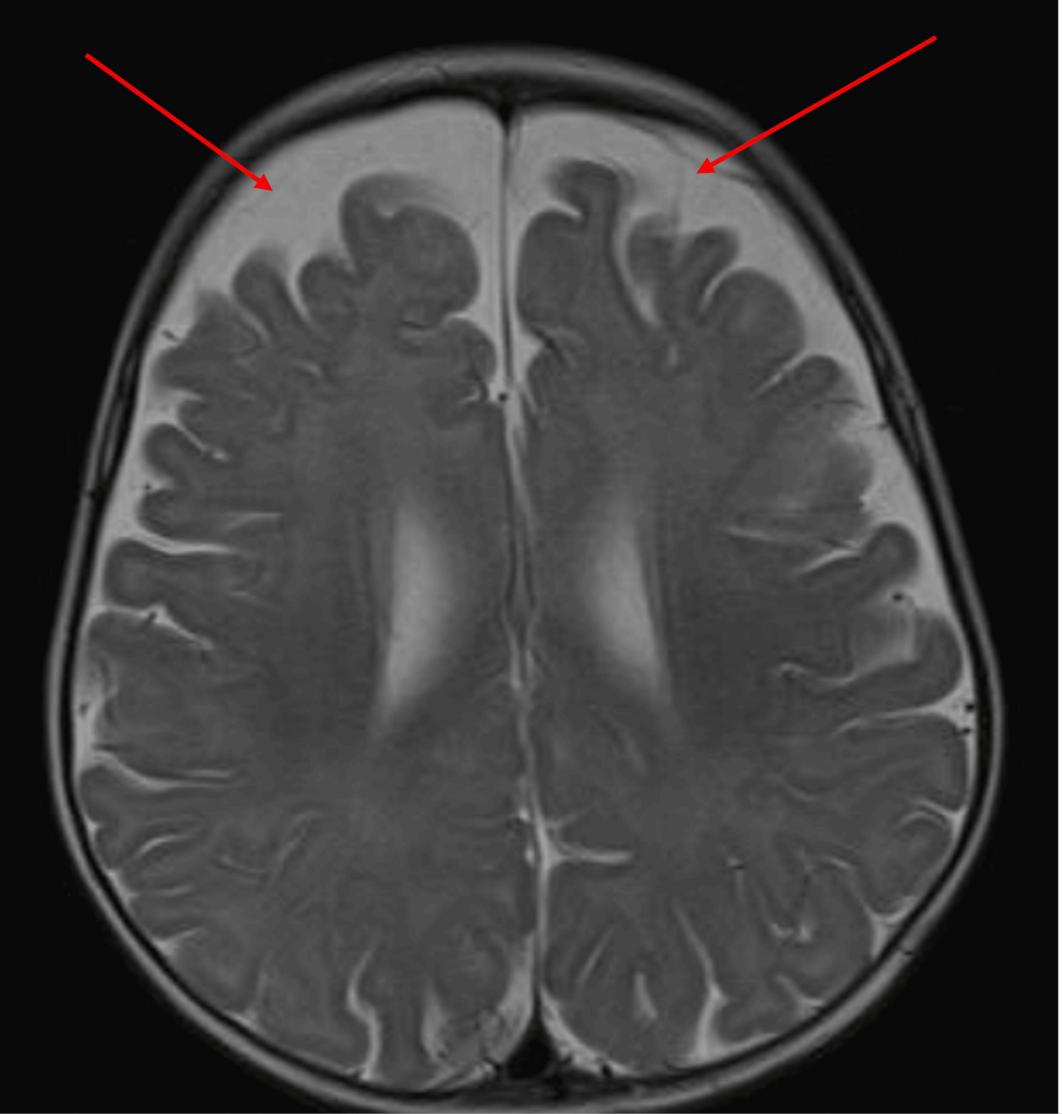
T2 sequence brain MRI - axial view The image shows increased EA-CSF volume in the subarachnoid spaces between the dura and cortical surface over the bilateral frontal lobes EA-CSF: extra-axial cerebrospinal fluid; MRI: magnetic resonance imaging
